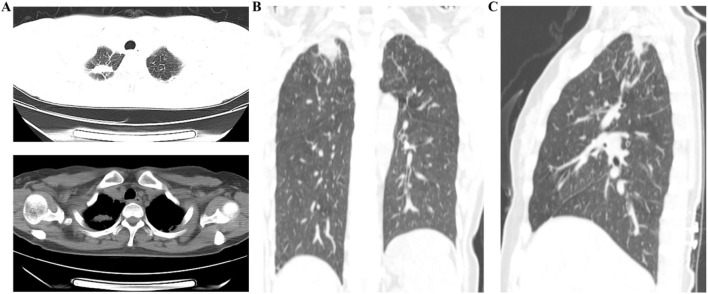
The results of the first plain chest CT scan after treatment. (A) Lung window and mediastinal window demonstrated a space-occupying lesion in the right upper lobe; (B,C) Coronal and sagittal plain chest CT images showed the space-occupying lesion in the right upper lobe.
